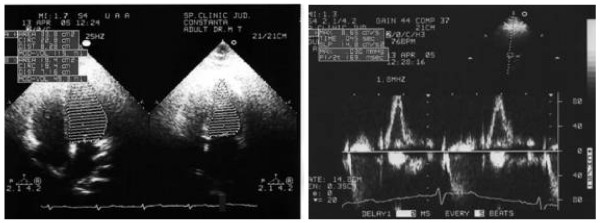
Patient 2. Echocardiography: left ventricular mass index, fractional shortening and fiber shortening velocity improved after 12 month of low-dose therapy with Re-GH.
